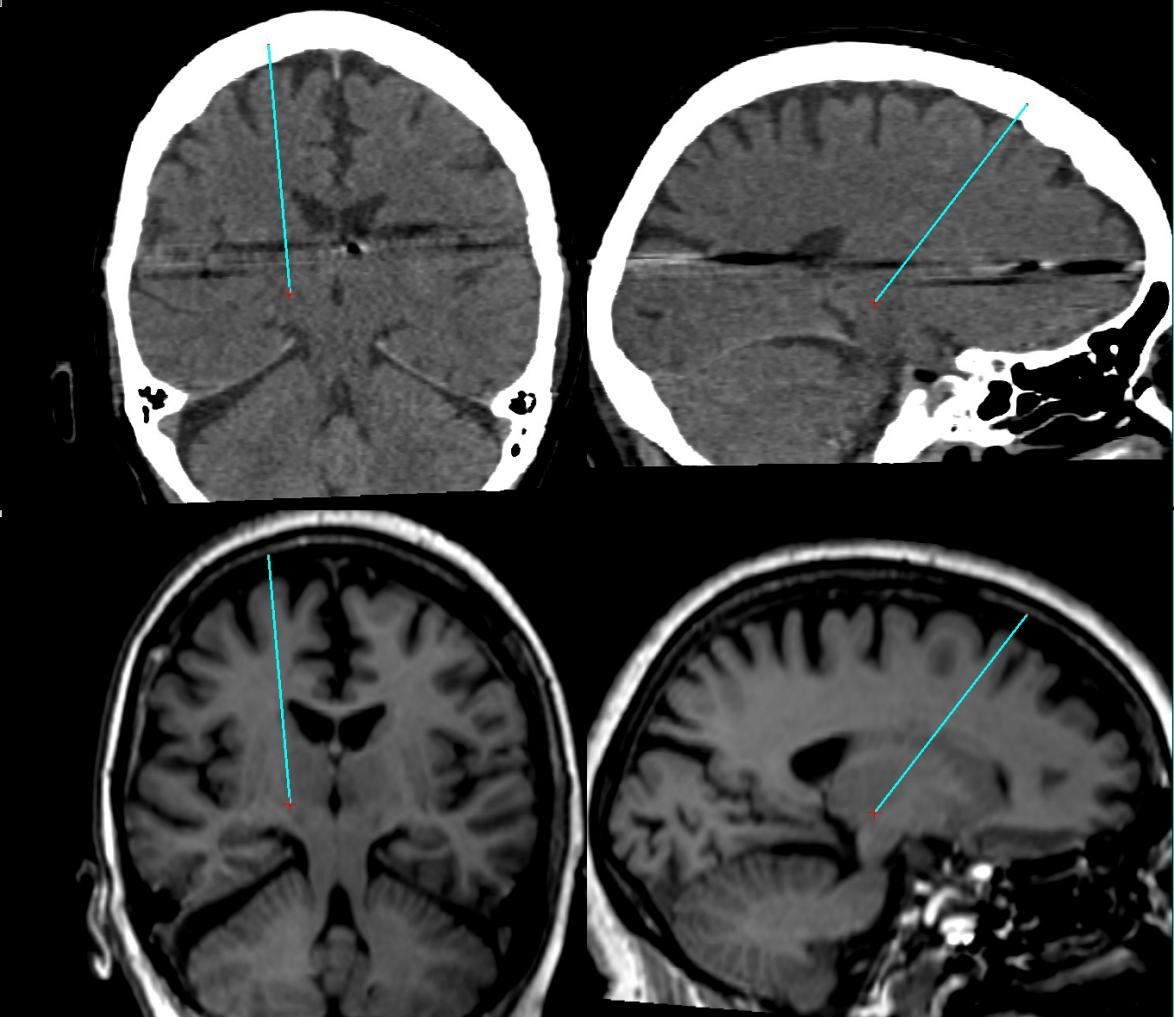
Probe insertion trajectory superimposed on para-coronal and para-sagittal images The target point depicted by the red cross and the entry point define a probe insertion trajectory depicted by the cyan line that is superimposed on para-coronal images (top left and bottom left) and para-sagittal images (top right and bottom right). These images are reconstructed from stereotactic computed tomography (CT) images (top left and top right) and from geometrically aligned, non-stereotactic magnetic resonance (MR) images (bottom left and bottom right). The metal tips of the Brown-Roberts-Wells (BRW) frame fixation screws produce artifacts in the stereotactic CT images. Compare to Figure 7.
